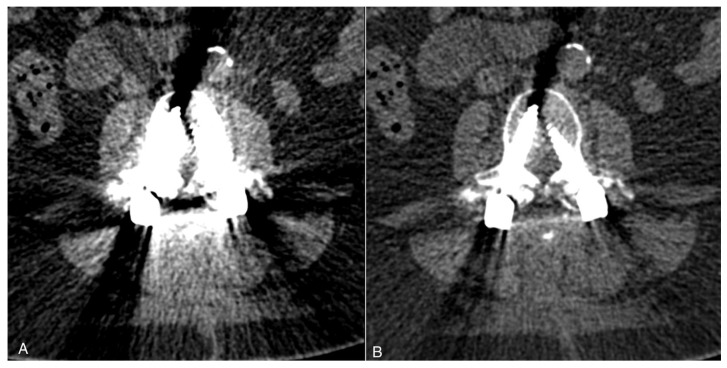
Effect of keV selection on metal artifact reduction in dual-energy CT. Axial images at L3 level in a 62-year-old woman with posterior instrumentation (L3–S1 pedicle screws and rods). (A) At 100 keV, a significant beam-hardening artifact obscures the hardware–bone interface. (B) At 140 keV, artifact burden is substantially reduced, improving visualization of pedicle screw-bone interfaces. (Reprinted with permission from reference [5].).
